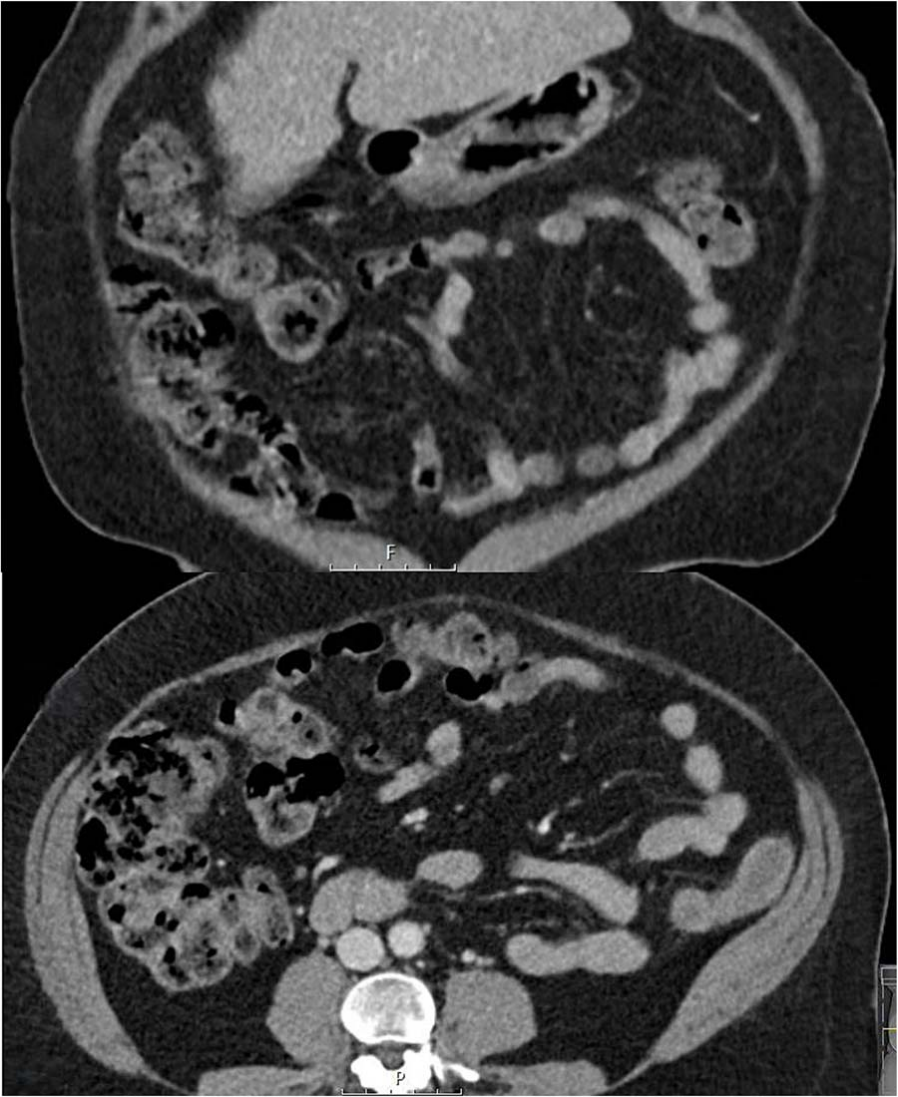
Follow up coronal and axial CT slices at 6 weeks showing resolution of PC.
